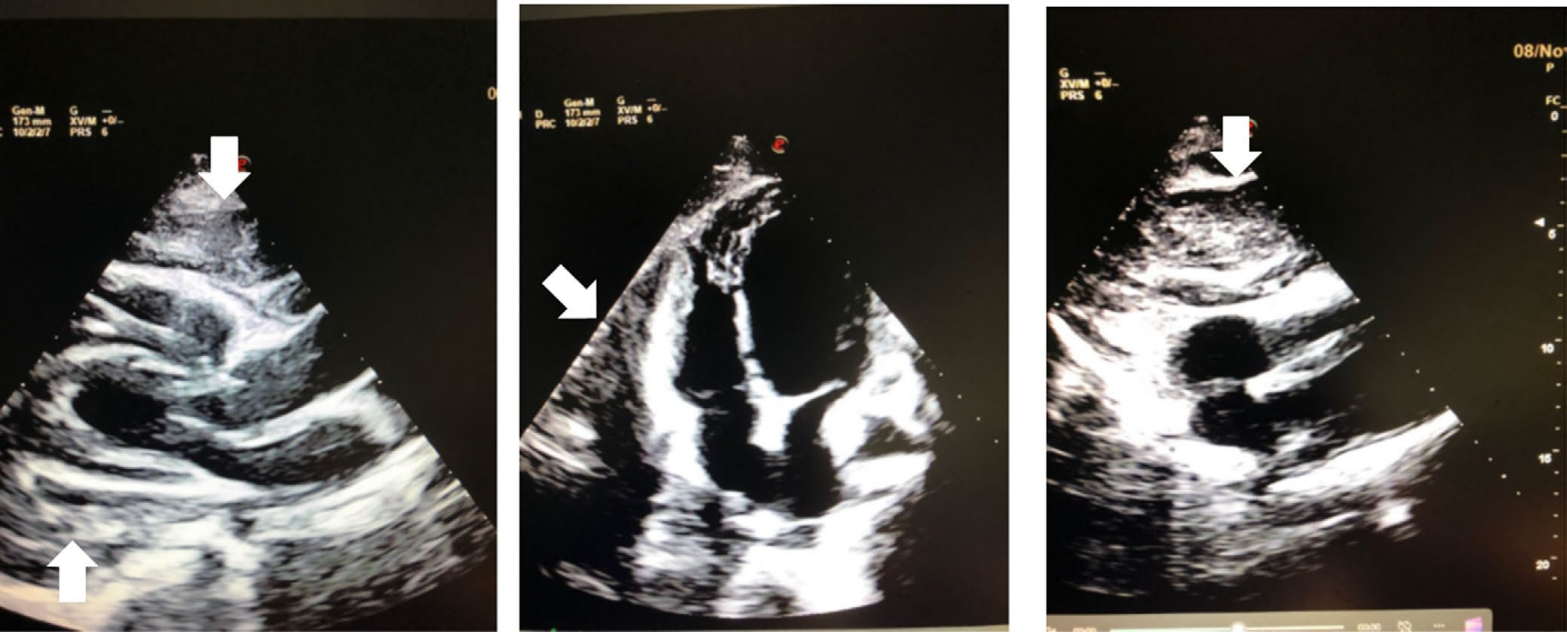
Admission TTE—left: Parasternal long axis view‐center‐apical 4 chamber view—right subxifoid view‐the heart is surrounded by not echo graphic homogenous space of maximal thickness 6 cm.
